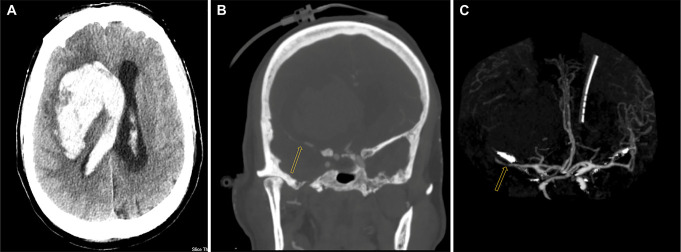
Initial CT/CTA before and after external ventricular drain insertion. A, The axial CT scan shows a right basal ganglia-originating hematoma extending into the ventricles with midline shifting, along with mild hydrocephalus. B, The CTA reveals a suspected large vessel occlusion/stenosis in the right MCA (indicated by an arrow). C, The CTA scan displays the hematoma and its proximity to the MCA, as well as how the hematoma and peripheral edema exert pressure on the MCA (indicated by an arrow). CT, computed tomography; CTA, CT angiography; MCA, middle cerebral artery.
